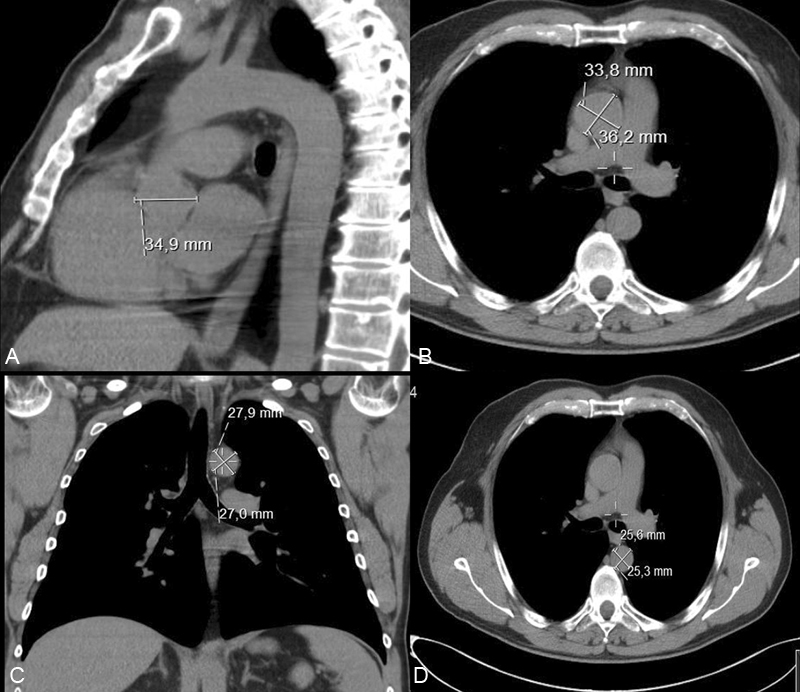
Thoracic aortic diameter measurements in low-dose chest computed tomography without intravenous contrast at (
